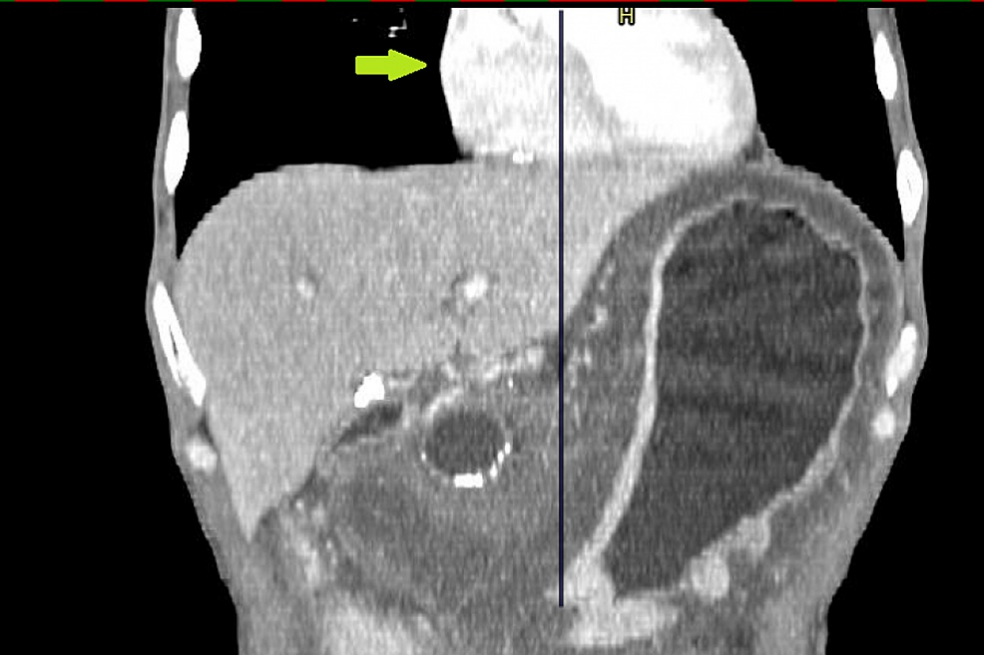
CT of coronal planes showing similar findings of absence of pericardium over the right heart (green arrow).
